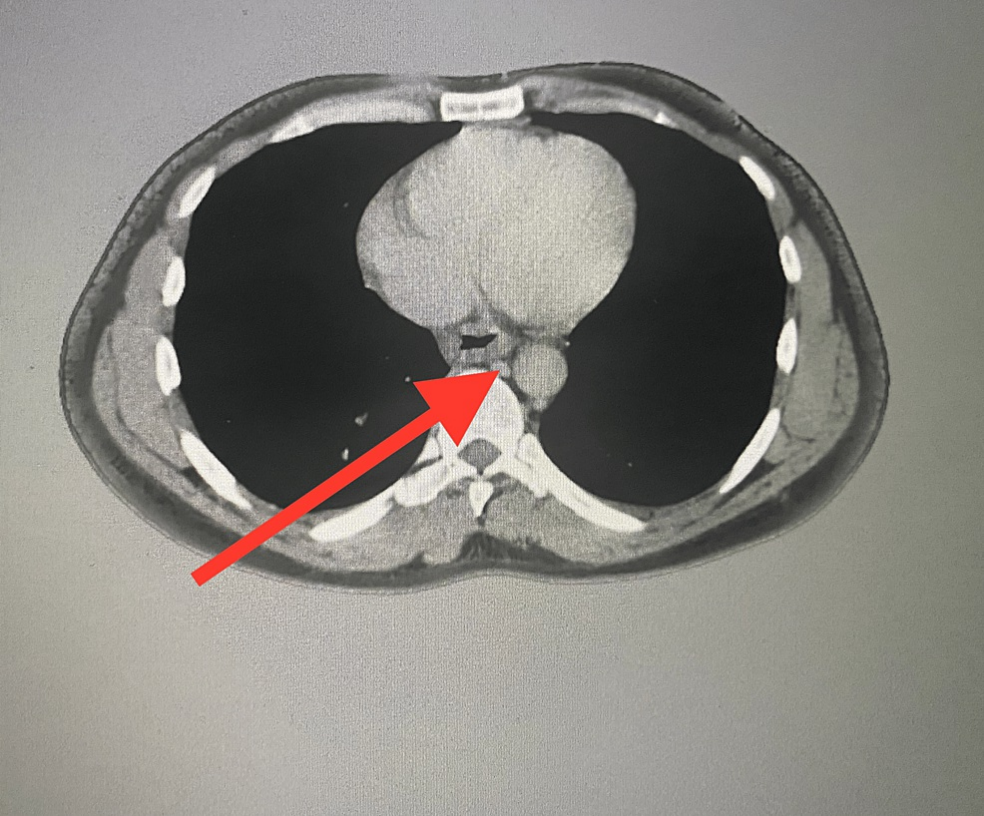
Computed tomography of the chest with contrast showed luminal narrowing of distal esophagus as shown with the arrow.
